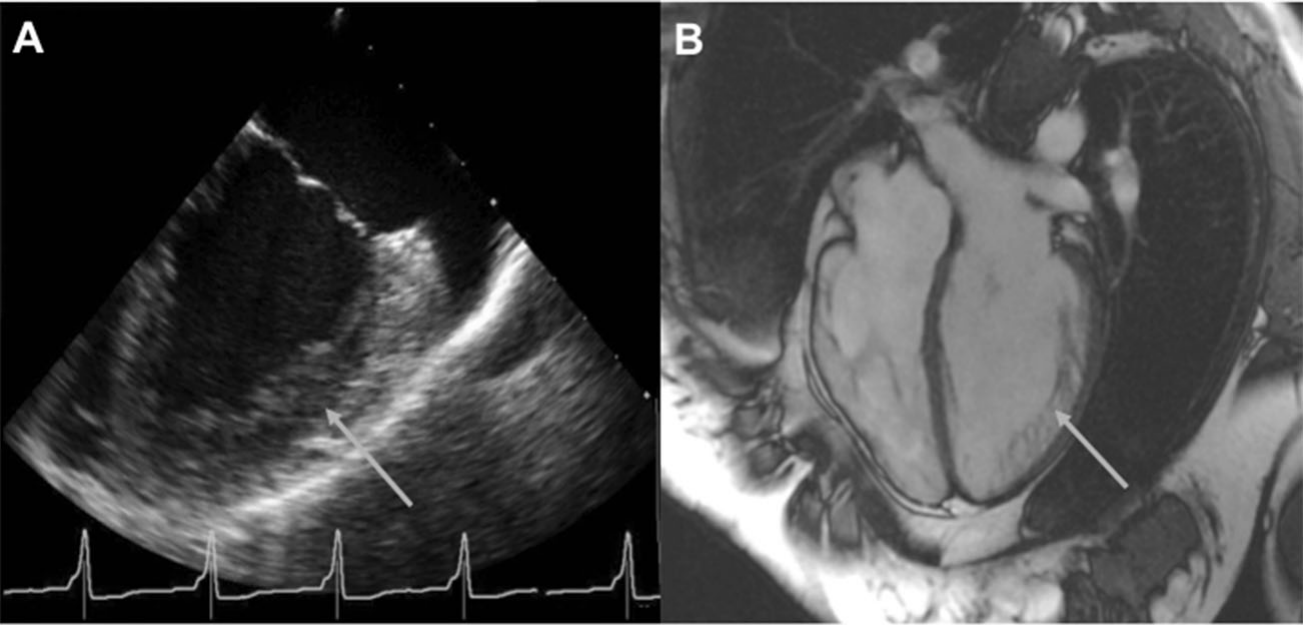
Cardiac imaging. Echocardiogram (a) and a magnetic resonance image (b) showing hypertrabeculation of the left ventricle (*arrow*)
